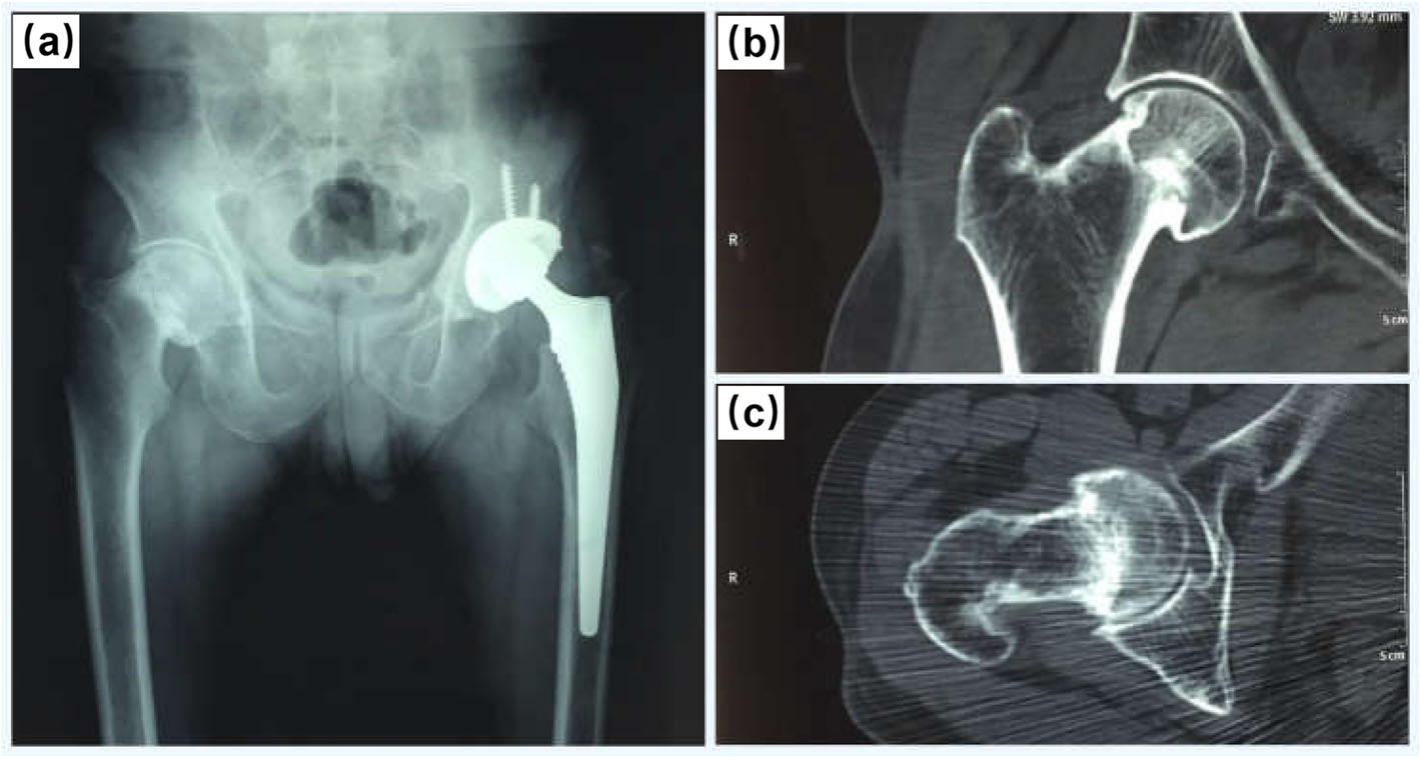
Imaging examination of the hip region for the patient four months after operation. a Radiograph of the pelvis showing well postoperative effect. b, c CT images of the right femoral neck showing fracture healing
